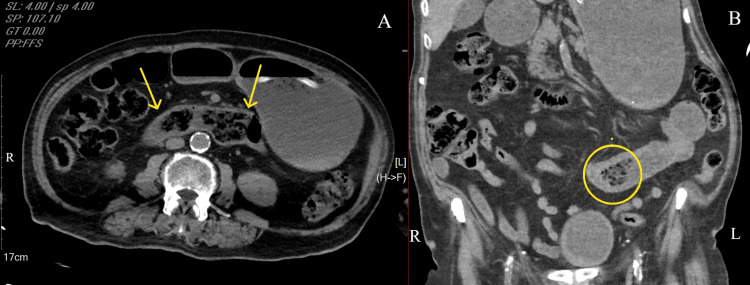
CT scan of the abdomen showing a phytobezoar at different levels of the jejunum. (A) Axial contrast-enhanced CT showing gastric distension and a mottled intraluminal mass in the proximal jejunum (phytobezoar indicated by yellow arrows). (B) Coronal CT image demonstrating a circumscribed jejunal phytobezoar (yellow circle) with mottled gas density resulting in upstream small bowel dilatation.
